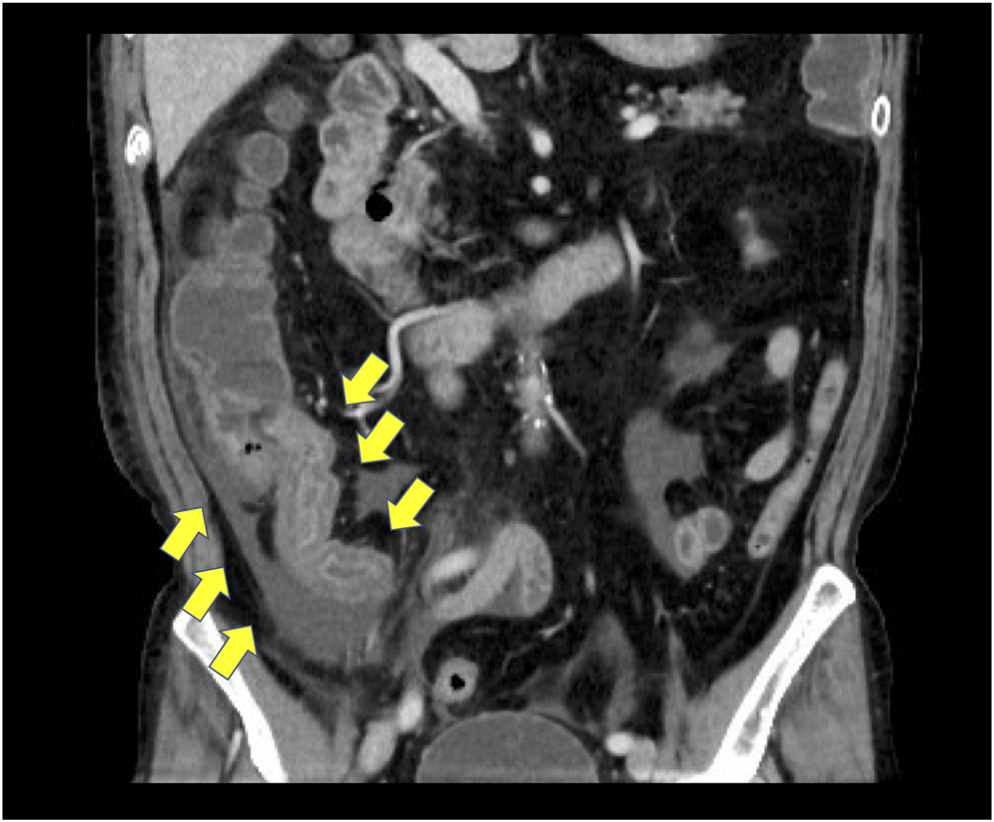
Contrast‐enhanced computed tomography showed that the terminal ileum wall was thickened, and ascites was observed around it. However, the contrast effect was relatively well preserved
